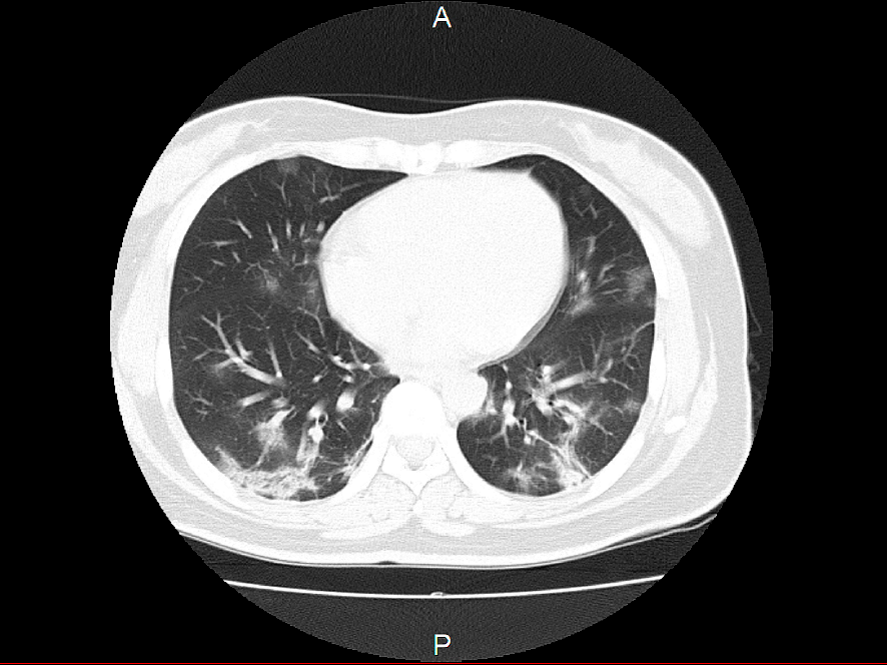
Chest CT image of a patient with coronavirus disease 2019 (a case diagnosed by the author).
